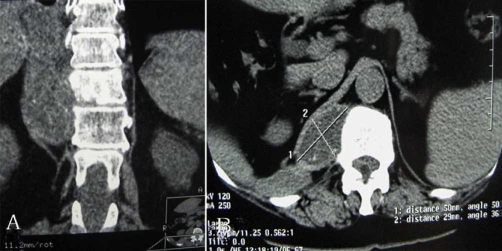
(A) Coronal CT scans showed that right lateral of paraspinal cystic lesions were involved in partial vertebra at the level of T9 12. The prominent destruction of T11 vertebra was visible. (B) Axial CT scans at the level of T11 showed a mass of 5 × 2.9 cm diameter was localized in the posterior mediastinum. The cyst has water density and at the posterior wall there are some foci of calcification.
